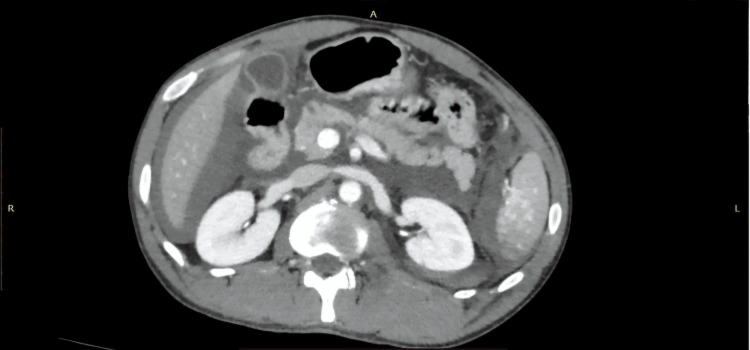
Simultaneous bilateral renal PCS opacification without extravasation on CECT (SB protocol). In the same patient (Figure 5), the use of the SB protocol resulted in simultaneous opacification of the bilateral renal pelvicalyceal system without any contrast extravasation. As a result, a delayed scan was not required, thereby reducing additional radiation exposure to the patient. PCS: pelvicalyceal system; CECT: contrast-enhanced computed tomography; SB: split-bolus.
